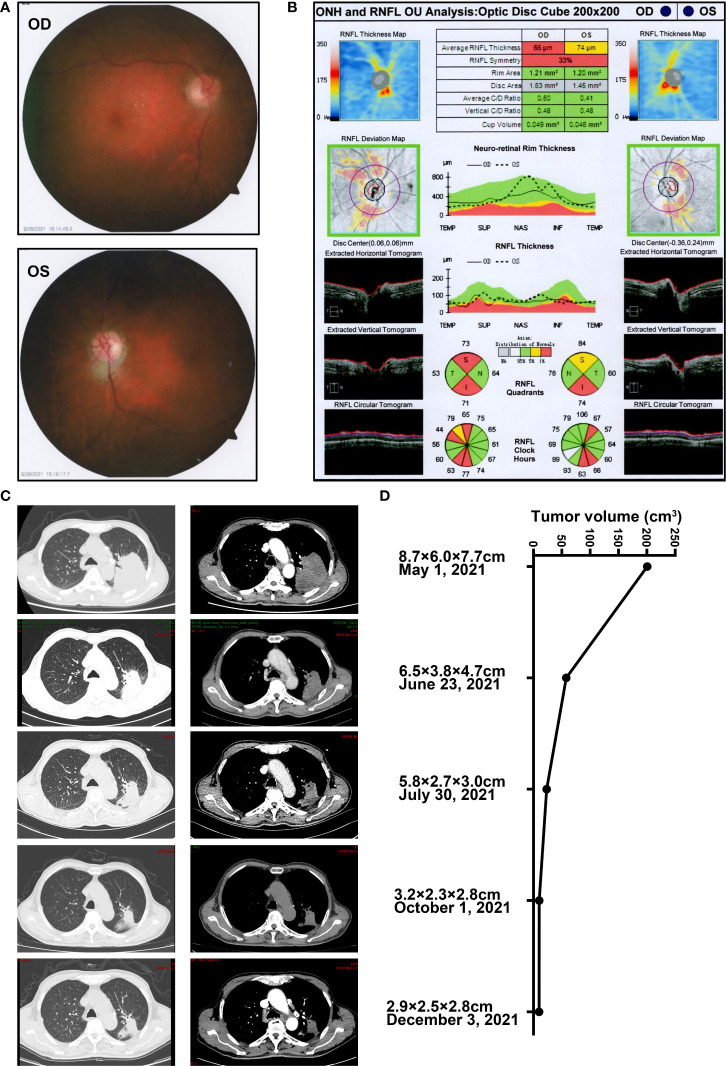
The ophthalmic examination of the patients post steroids against sintilimab-induced optic neuropathy and representative CT images in the follow-up (A) Fundus photography post steroids against optic neuropathy secondary to sintilimab therapy on 28 September 2021. (B) OCT examination of the optic disc post steroids against sintilimab-induced optic neuropathy on 28 September 2021. (C) Representative CT images and (D) the estimated tumor volume during the treatment of the case in the follow-up from 1 May 2021, to 3 December 2021. The horizontal axis represents changes in tumor volume. The vertical axis depicted the time of CT scan and the estimated tumor longitudinal diameter (X) and the greatest transverse diameter (Y) in the axial plane and the maximum thickness in head-to-foot direction (Z). The tumor volume was calculated by using the formula (X*Y*Z)/2.
